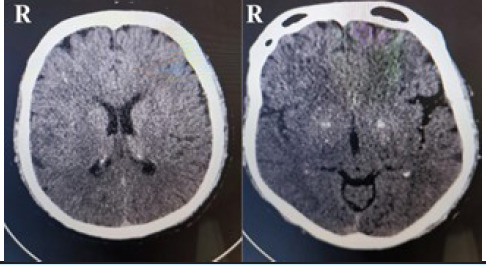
Non-contrast enhanced computed tomography (NCCT) showed no acute abnormalities.
